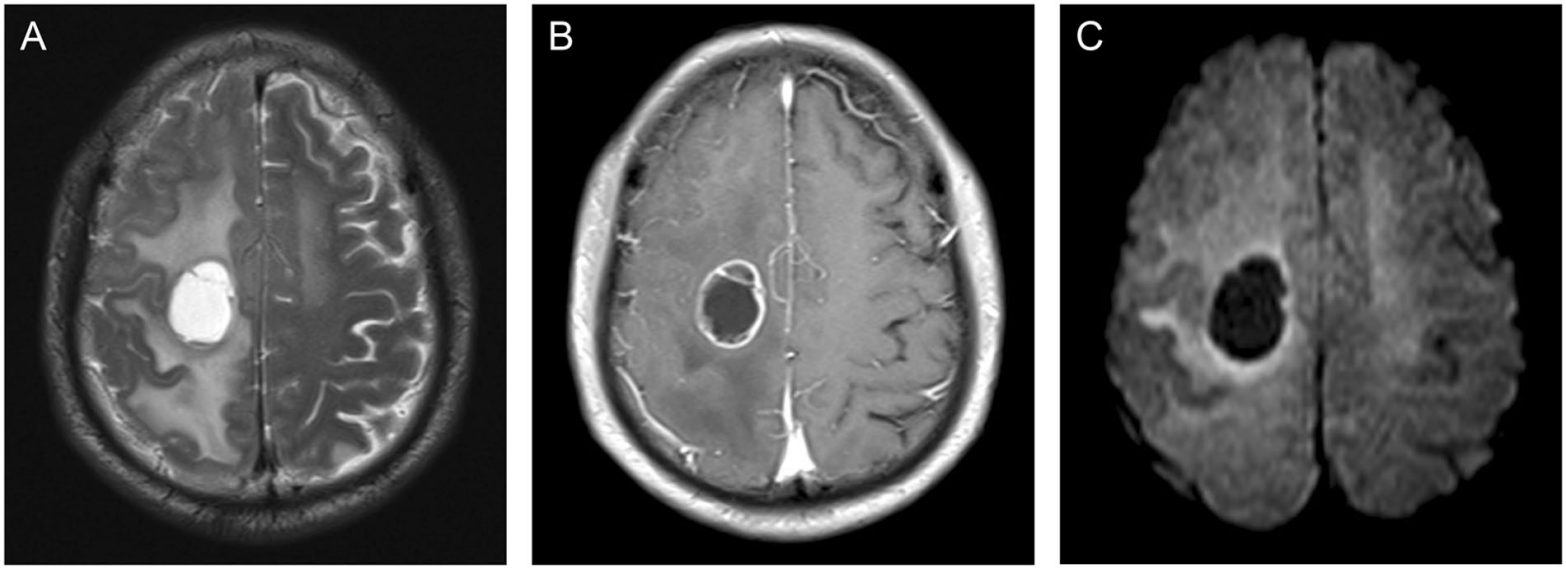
A right frontal lobe Grade III anaplastic PXA in a 51-year-old male with a predominantly cystic mass without an obvious mural nodule. (A) Axial T2WI showing the iso-intense cystic wall and homogeneous hyper-intense appearance of the cyst-like area. Obvious vasogenic edema surrounded the mass. (B) Contrast-enhanced axial T1WI showing the cystic wall with intense enhancement and the cyst-like area without enhancement. (C) DWI showing no evidence of restricted diffusion of the tumor.
